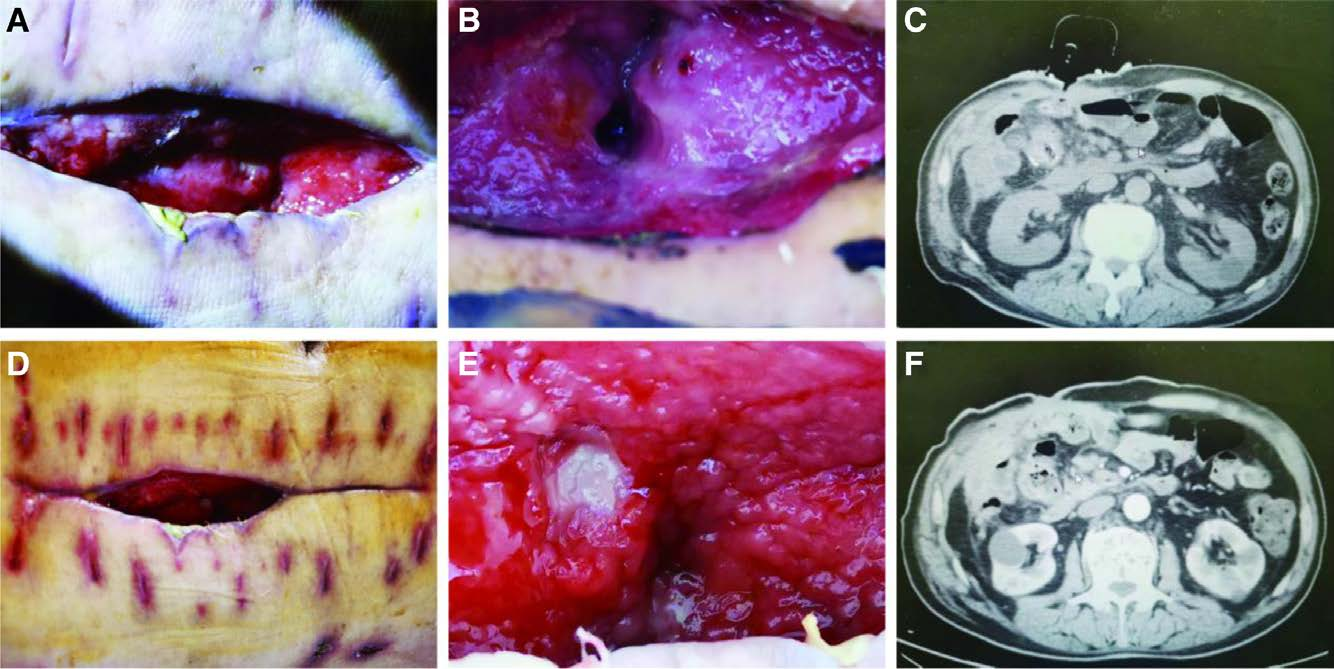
Incision, intestinal leakage, and CT after enterocutaneous fistula. (A) Necrotic tissue at the bottom of the incision was replaced with fresh granulation tissue. (B) The intestinal leakage was significantly narrower than the previous leakage. (C) Granulation tissue growth and narrowing of fistula tract. (D) Further narrowing of the incision. (E) Intestinal leakage healed after treatment and the incision was lined with fresh granulation tissue. (F) Intestinal leakage healed, and the fistula tract was completely filled with fresh granulation. CT = computed tomography.
